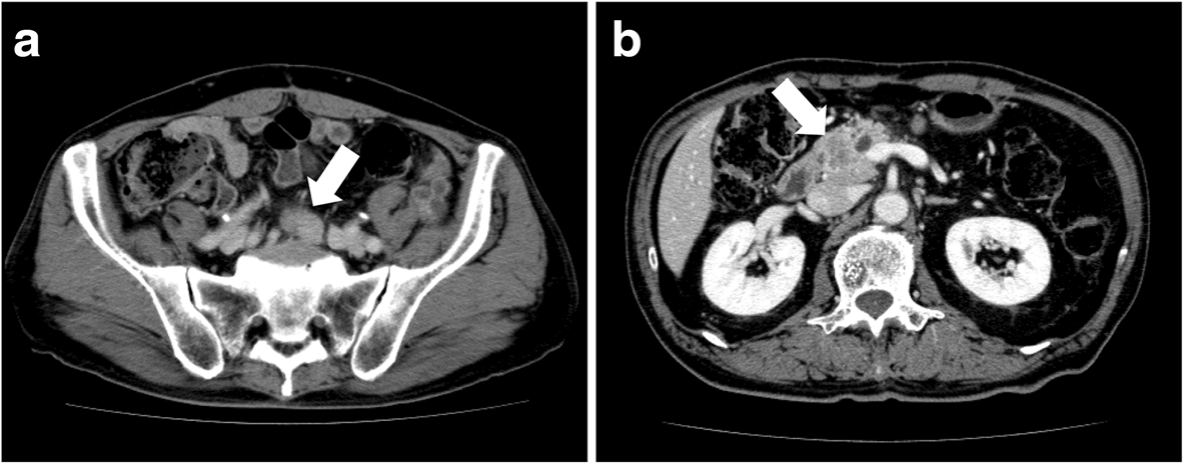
Preoperative findings of abdominal contrast CT. a An enlarged retroperitoneal lymph node (28 mm in diameter) is confirmed below the aortic bifurcation (*arrow*). b A hypovascular tumor (25 mm in diameter), which was growing invasively, is shown in the head of the pancreas (*arrow*)
